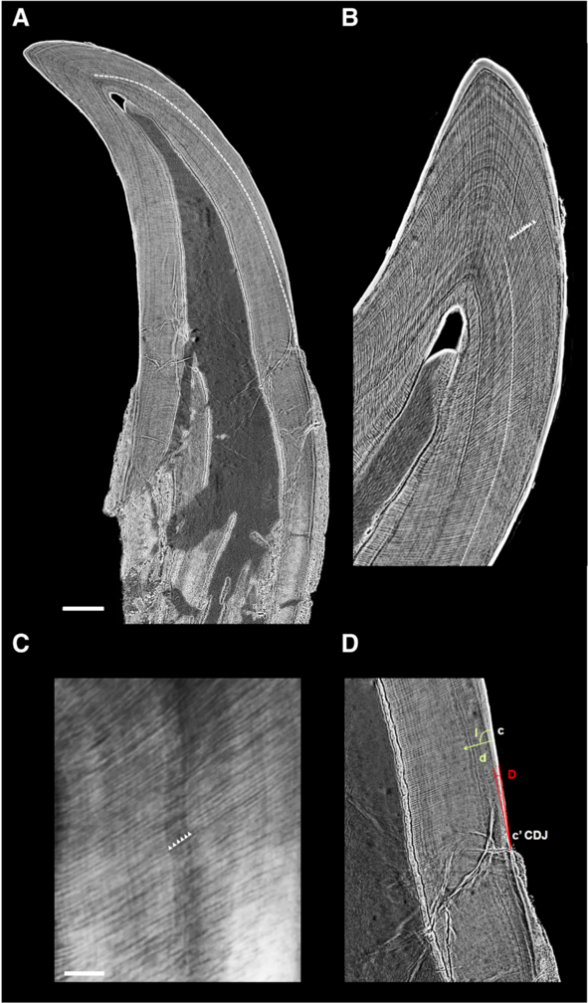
Synchrotron x-ray microtomographic images of *Hesperornis* tooth dentine increment lines (YPM.1206.B). a Parasagittal basal-apical section of the *Hesperornis regalis* isolated tooth YPM.1206.B, showing its dentine increment lines. b Higher magnification of the apex of the tooth from the same section. Regular incremental lines are observed throughout the dentine (white arrows), and are interpreted as daily lines. They are counted from the dentine horn to the incremental line corresponding to crown completion (dashed line). c Higher magnification of the series of incremental lines observed in the dentine and interpreted as daily increments. d Higher magnification at the cervix level of the area near the cementum-dentine junction (CDJ) in the root, for quantifying the tooth root extension rate. d, direction of the daily rate of dentine secretion (DSR); angle I, angle the dentine tubules make with the root surface; angle D, angle between an incremental line and the root surface. Scale bars a 0.5 mm, c 0.05 mm
